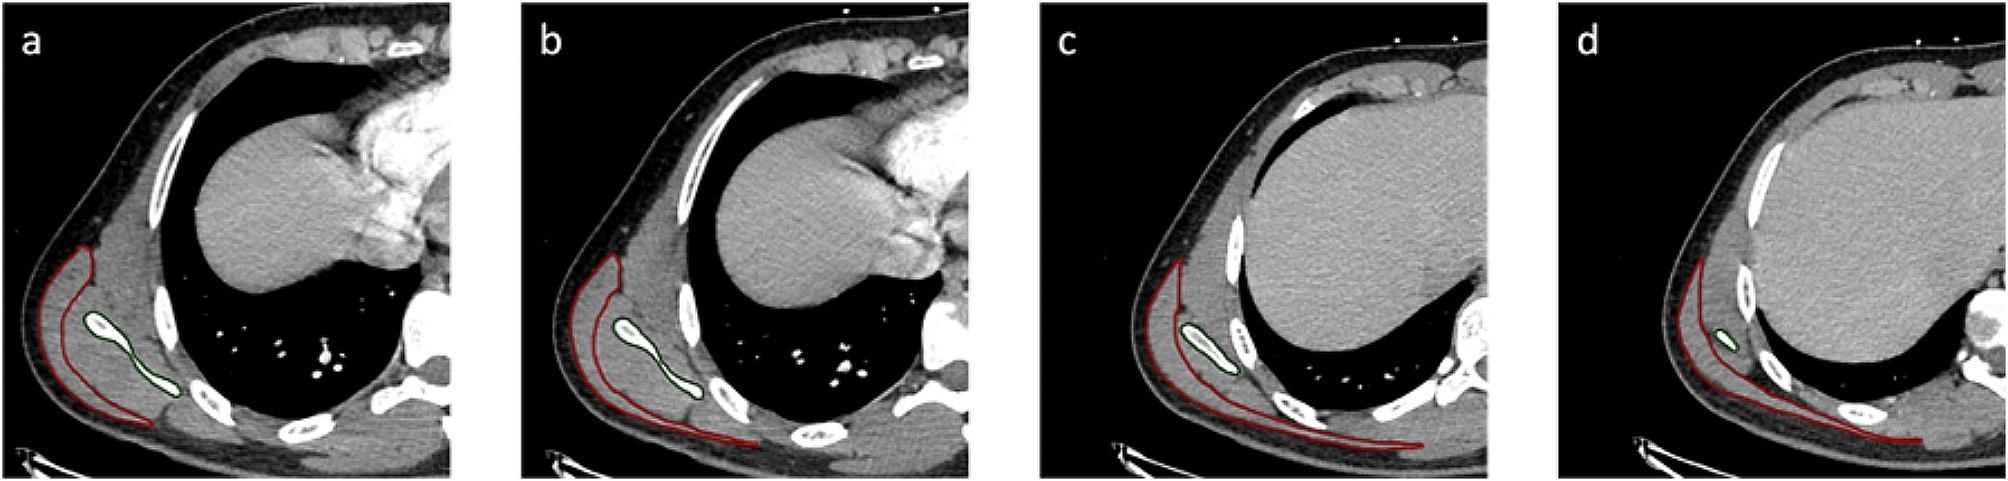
Exemplary axial CT views from cranial to caudal direction (a$$\to$$d) of a right shoulder in arms up position. The regions of the scapula covered by the latissimus dorsi muscle are depicted in green, and the borders of the latissimus dorsi muscle in red
